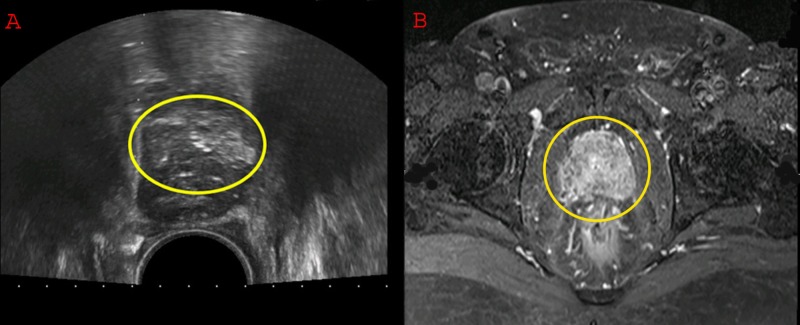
(A) Ultrasound of the pelvis showing an enlarged prostrate. (B) Magnetic resonance imaging (MRI) of the pelvis with contrast showing an enhancing lesion in the prostrate.
